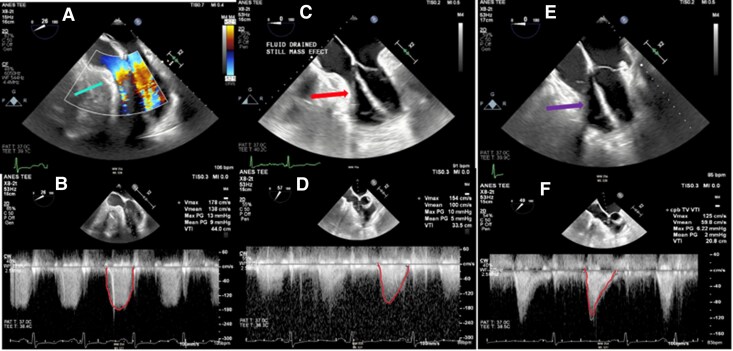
Intraoperative transoesophageal echocardiography (TEE) before and after pericardial interventions. Prior to drainage, a heterogeneous pericardial fluid collection (arrow, Panel *A*) was seen adjacent to the right-sided chambers with compressive effect, accompanied by an elevated tricuspid mean diastolic gradient of 9 mmHg at 105 bpm (Panel *B*). After drainage, the compressive mass effect persisted (arrow, Panel *C*) with only partial improvement in haemodynamic, as the tricuspid mean gradient remained elevated at 5 mmHg at 88 bpm (Panel *D*). Following pericardiectomy, there was clear decompression of the right-sided chambers (arrow, Panel *E*) with normalization of haemodynamic, and the mean diastolic gradient reduced to 2 mmHg at 83 bpm (Panel *F*).
